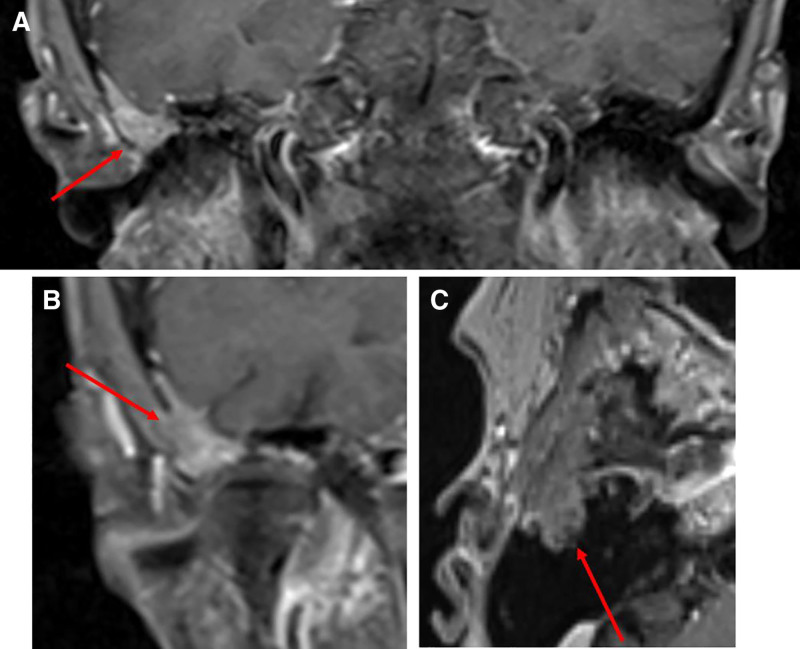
MRI without contrast. A, B, Coronal images, red arrow indicates the area of enhancing lesion within the temporal bone. C,. Axial image, red arrow indicates the area of enhancing lesion.
